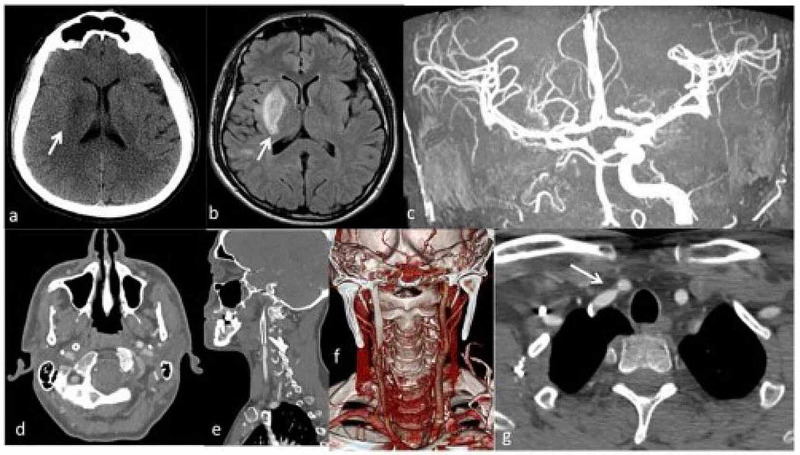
Computed tomography imaging performed after one-week follow-up The one-week follow-up computed tomography (a) showed a hypodense signal in the region of right basal ganglia (arrow) confirmed by T2w fluid attenuation inversional recovery (arrow) consistent with ischemic subacute damage. The maximum intensity projection in coronal plan (c) of time of flight sequence showed good recanalization of the right middle cerebral artery with the signal in the internal carotid artery (arrowhead). The arterial phase of computed tomography (d) confirmed the occlusion of the stent (arrowhead) showing also in the sagittal plane (e) and volume rendering imaging (f). As collateral findings, severe stenosis of the right subclavian artery (arrow) was found (g).
